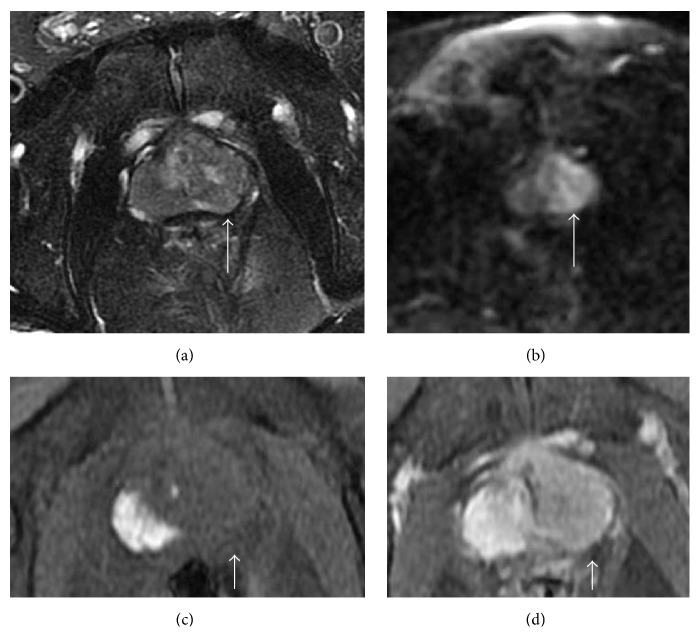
On MRI, T1 weighted image showed diffusely spread tumor in the left side of the prostate (white arrow). This lesion was heterogeneous on T2 weighted images (a), high intensity on diffusion weighted images (b), and well enhanced by contrast agent ((c) T1 weighted image, (d) contrast-enhanced T1 weighted image).
